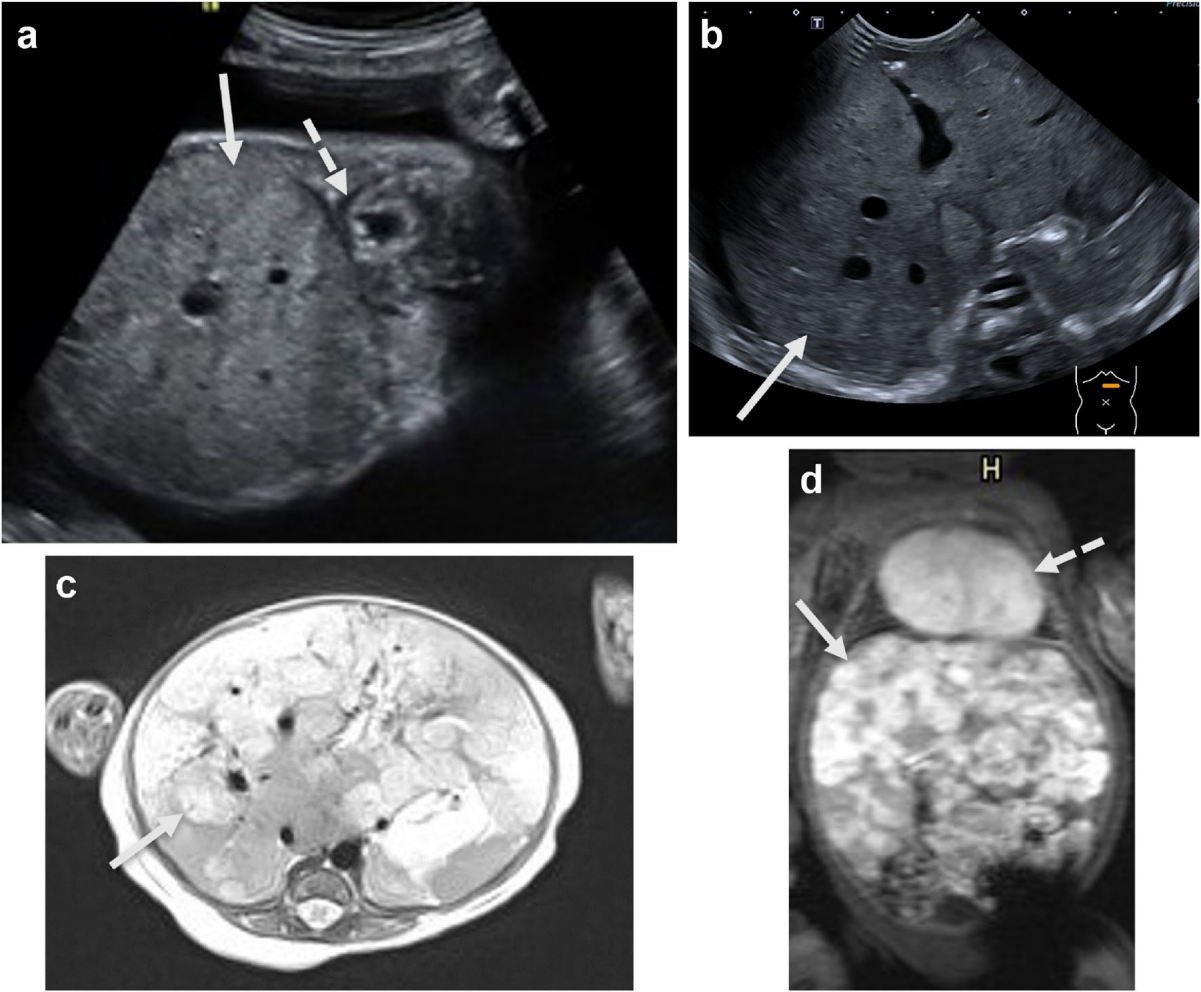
Congenital hemangiomas of the liver in a female fetus and neonate. a Prenatal coronal ultrasound at 30 weeks gestational age, demonstrates an enlarged inhomogeneous liver (*arrow*) and some pericardial fluid (*broken arrow*). Additionally, cardiomegaly, multiple skin lesions and some ascites were identified (not shown). The liver abnormalities were consistent with the diagnosis of hepatic hemangiomatosis. b–d Postnatal transverse ultrasound (b), axial T2-weighted magnetic resonance (c)  and coronal T1-weighted postcontrast magnetic resoance (d) images after delivery at 32 weeks gestational age, demonstrate multiple nodular liver lesions (*arrows*) with high vascularity, causing extensive shunting and cardiomegaly (*broken*
